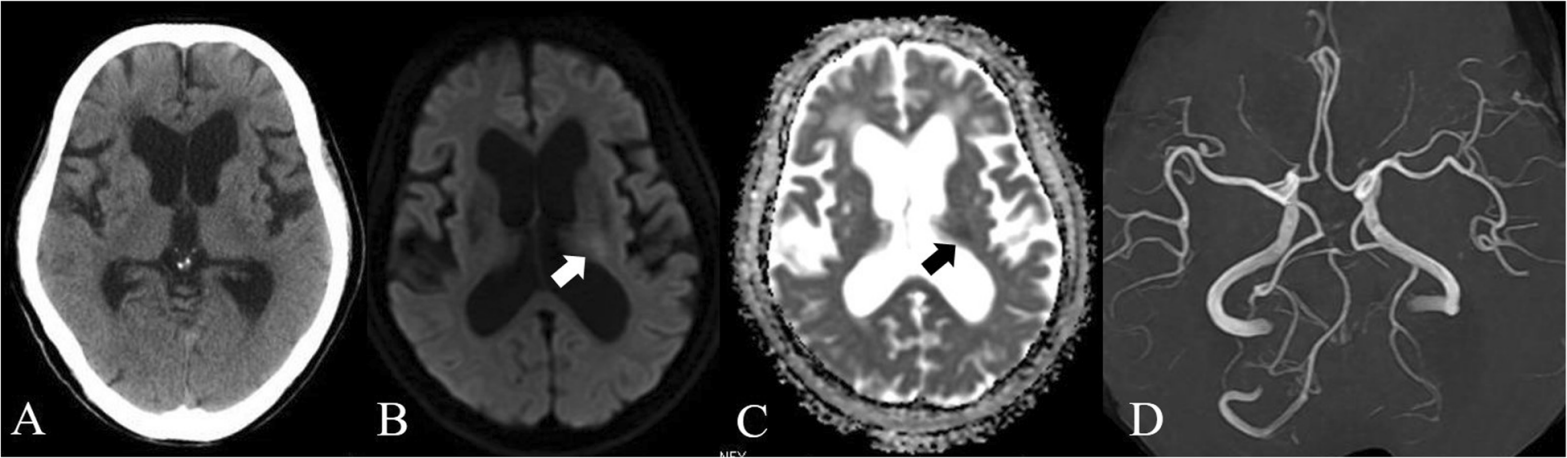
Images imported to our emergency center upon admission. Head computed tomography immediately after our emergency center import and prior to administration of idarucizumab showing a small, low-density spot in the right putamen due to old lacunar infarct, and no cerebral hemorrhage (a). Mild high-intensity signals on diffusion-weighted magnetic resonance (MR) imaging revealed the left internal capsule (b; *white arrow*) and reduced apparent diffusion coefficient (c; *black arrow*). MR angiography showed no large-vessel occlusion (d)
